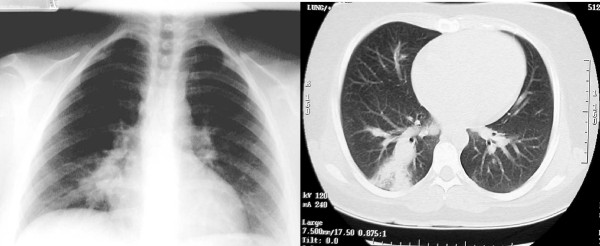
Chest radiograph revealed an ill-defined rounded infiltrate in the right lower lobe (white arrow).
